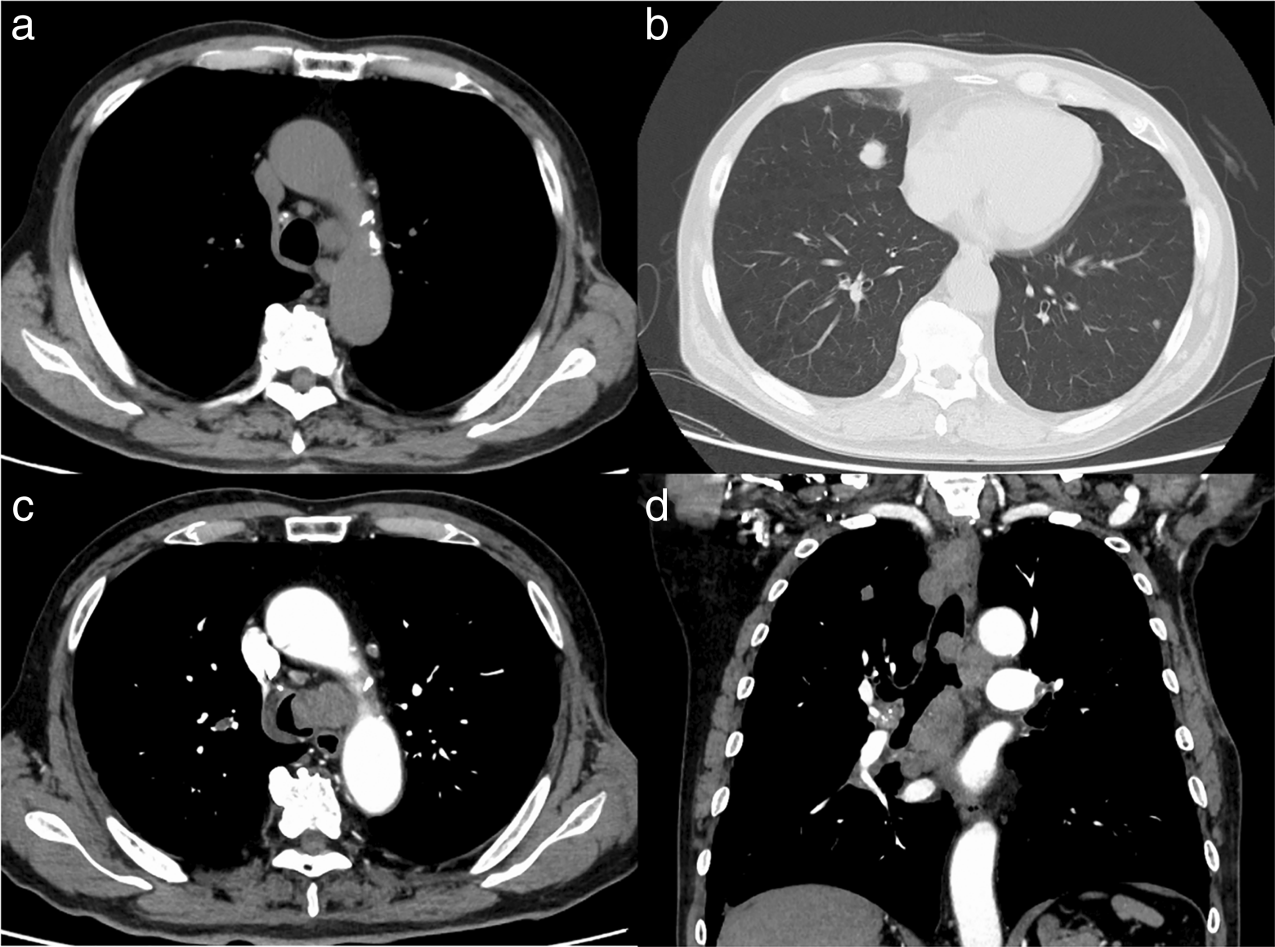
Chest computed tomography (CT) on the initial visit and admission. (a, b) Chest CT on the initial visit showing multiple pulmonary nodules in both lungs and an enlarged lower paratracheal (no. 4 L) lymph node (LN) (a, b; axial image). (c, d) Chest CT on admission showing airway stenosis and a connected tracheal tumor from the bronchoscopically biopsied LN into the tracheal lumen in addition to further mediastinal LN enlargement (c; axial image, d; coronal image)
